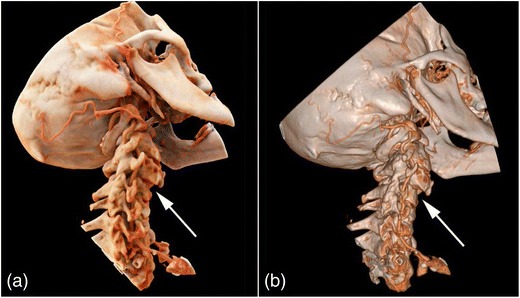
Contrast-enhanced 3-D CT images of a cervical spine injury with luxation of C3/4. Both CR (a) and VR (b) images show the anterior subluxation of the cervical vertebrae (arrows). The enhanced lightning of CR creates a high contrast between vessels and bone, making it easy to follow the course of the vertebral artery
